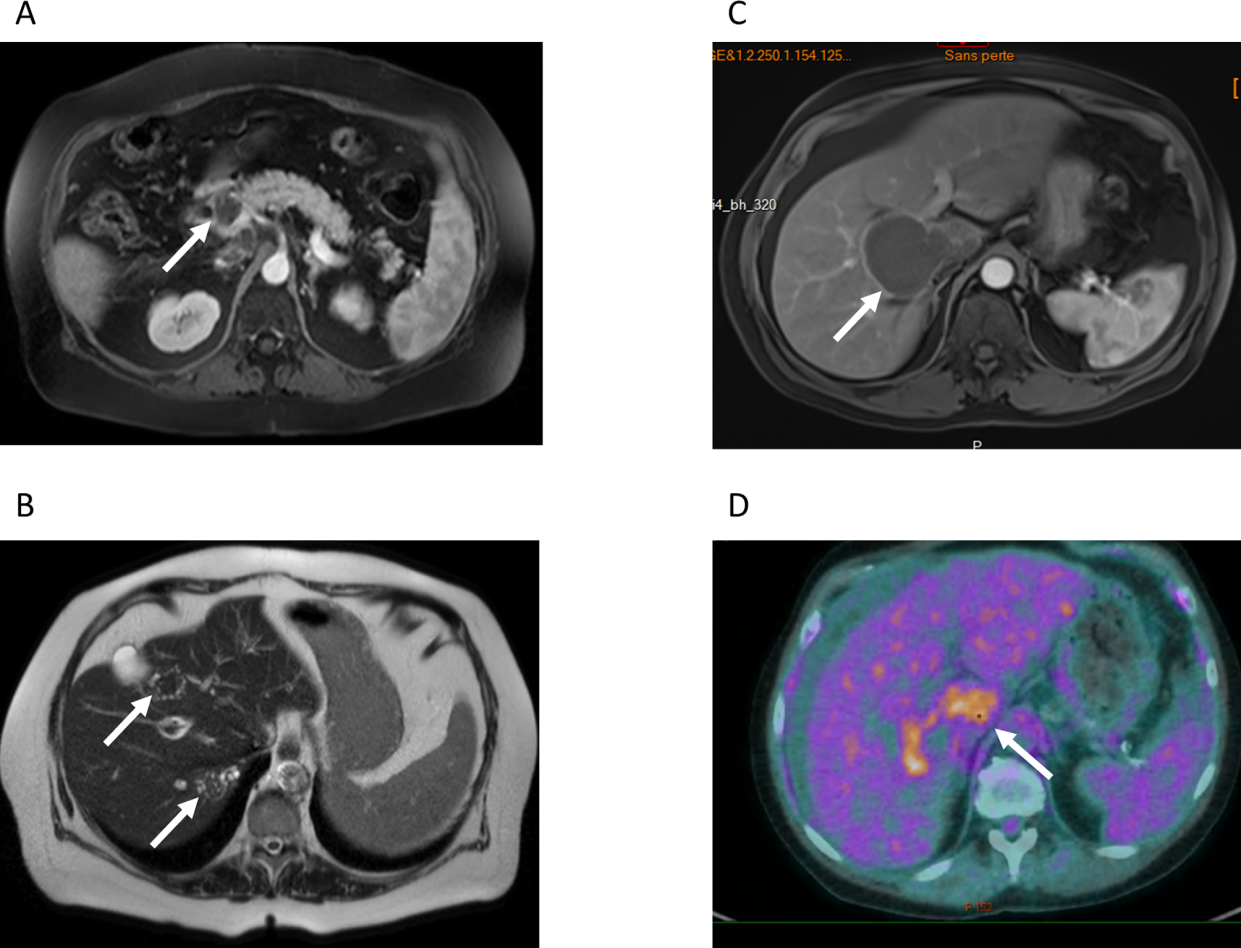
Radiological findings. *A) and B) MRI of patient #5 A) T1-weighted MRI of patient #5. White arrow: lesion in the head of the pancreas. This lesion was mistakenly interpreted as pancreatic cancer. B) T2-weighted, the white arrows point at the typical microcysts in T2-hypersignal. C) T1-weighted MRI of patient #4, showing a large well-delimited lesion tumor-like lesion, with invasion of the right portal branch D) PET-CT of patient #4, showing intense metabolic activity at the periphery of the lesion.
